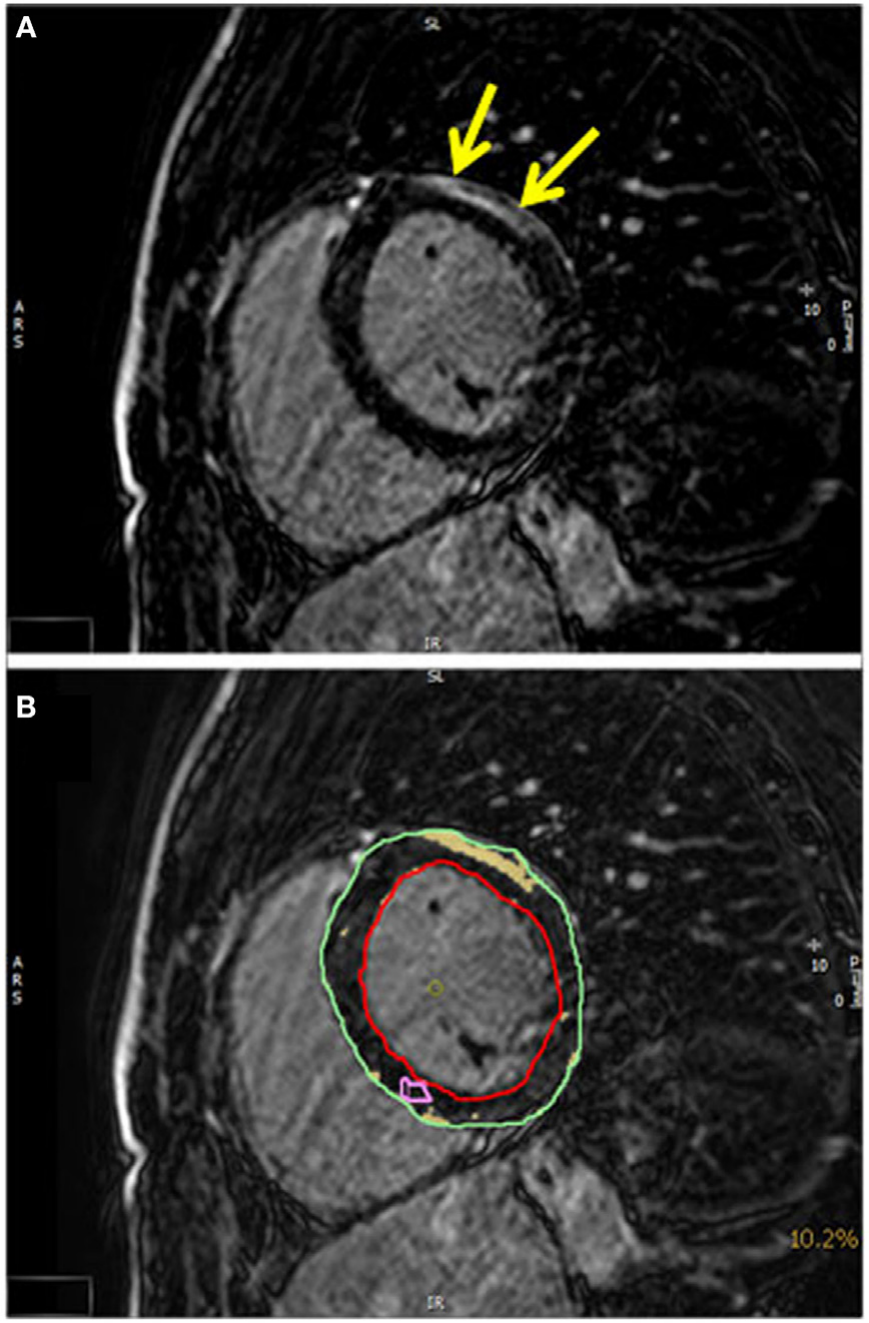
(A) A T1-weighted inversion-recovery sequence in short axis view showing epicardial late enhancement in the anterolateral myocardial segments (yellow arrows). (B) The quantification of the late enhancement area (yellow) in relation to the myocardial mass (epicardial contour green and endocardial contour red).
